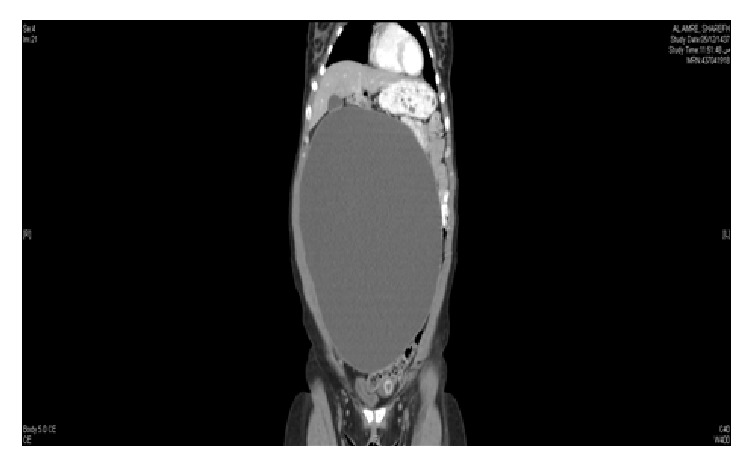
Abdominopelvic computed tomography (CT) showing a large well-defined homogeneously cystic lesion originates from the right ovary measuring 15.6 × 26.3 × 31.7 cm.
